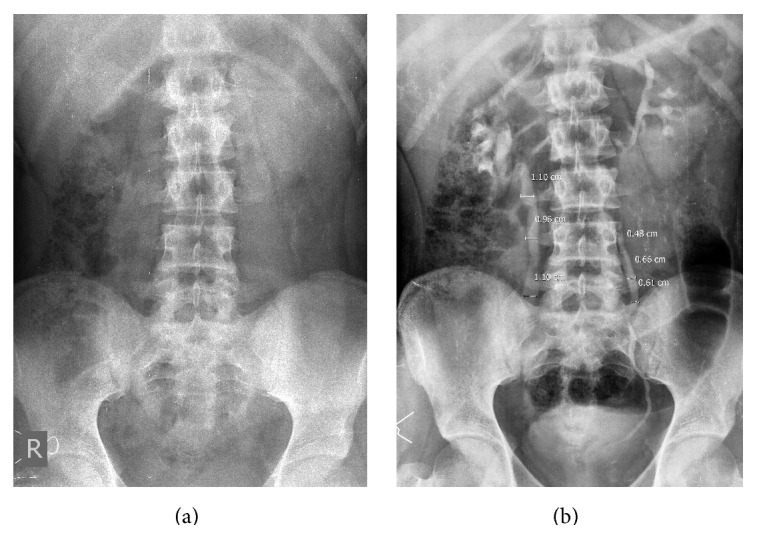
(a) Scout film: male patient with right-sided flank pain. No radiopaque calculus seen. (b) IVU: right ureter was dilated, but the cause was not obvious. No vesicoureteral reflux seen on color Doppler. Urine examination revealed tuberculosis.
